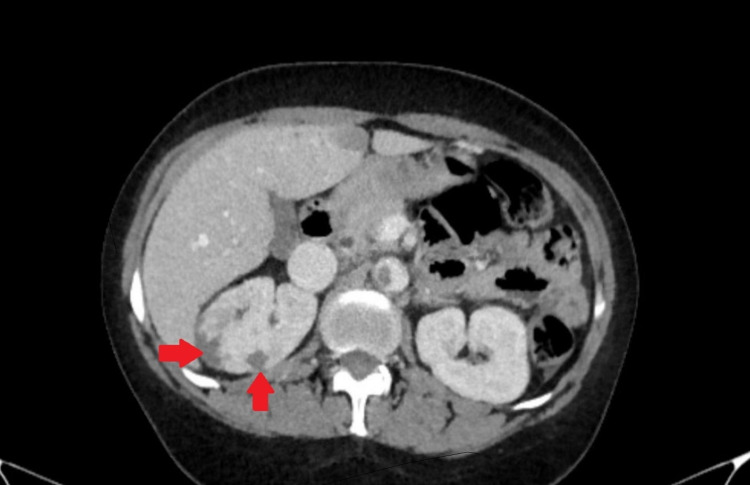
CT abdomen showing wedge-shaped hypodensities in right kidney.
